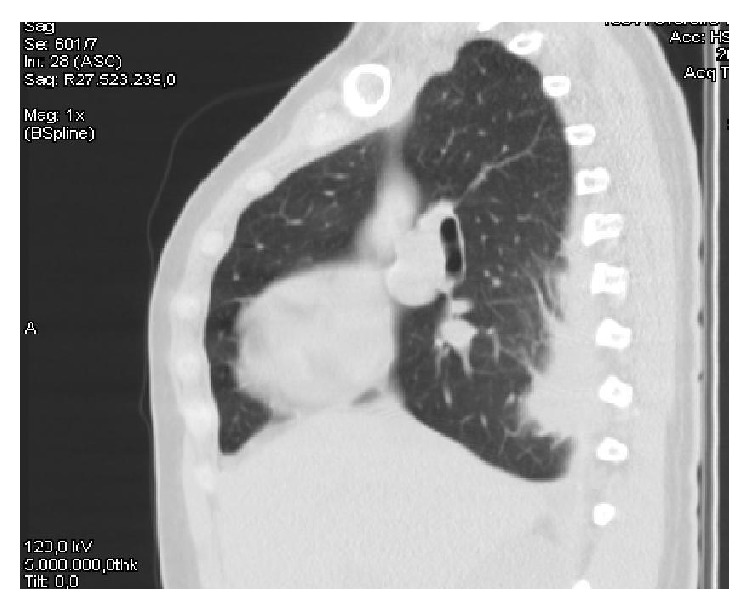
Chest CT with evidence of pleural right lesion.
